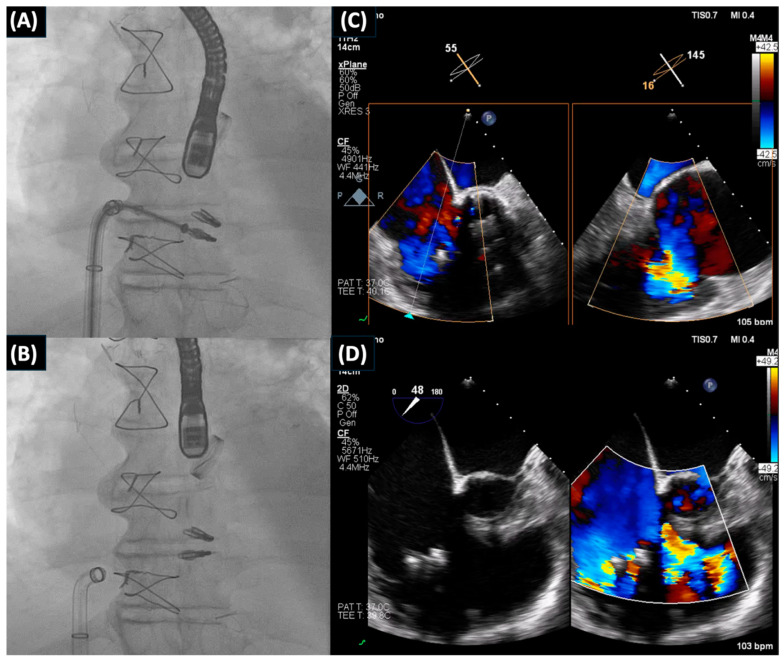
A 81 year old man presented with symptoms of RV heart failure and torrential tricuspid regurgitation. He was under optimal medical therapy. Echocardiography subsequently demonstrated torrential tricuspid regurgitation with posterior leaflet flail. After Heart Team review, the patient underwent transcatheter tricuspid valve repair with the TriClip™ system (Abbott Vascular, Santa Clara, CA, USA). (A,B): Transcatheter tricuspid valve repair with the TriClip™ system. (C,D): Intra-procedural transoesophageal echo revealing the torrential tricuspid regurgitation and assisting the operators for the optimal implantation of the clips.
